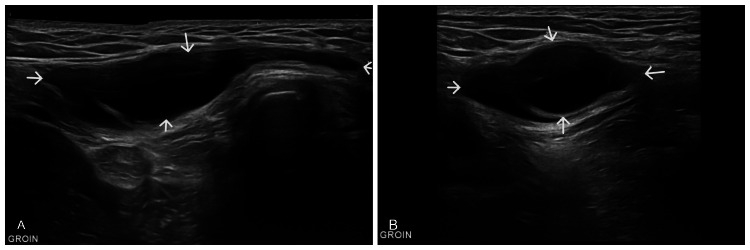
Ultrasound scan showing an anechoic cystic tubular structure (arrows) in the right groin measuring 64x15x36mm.
